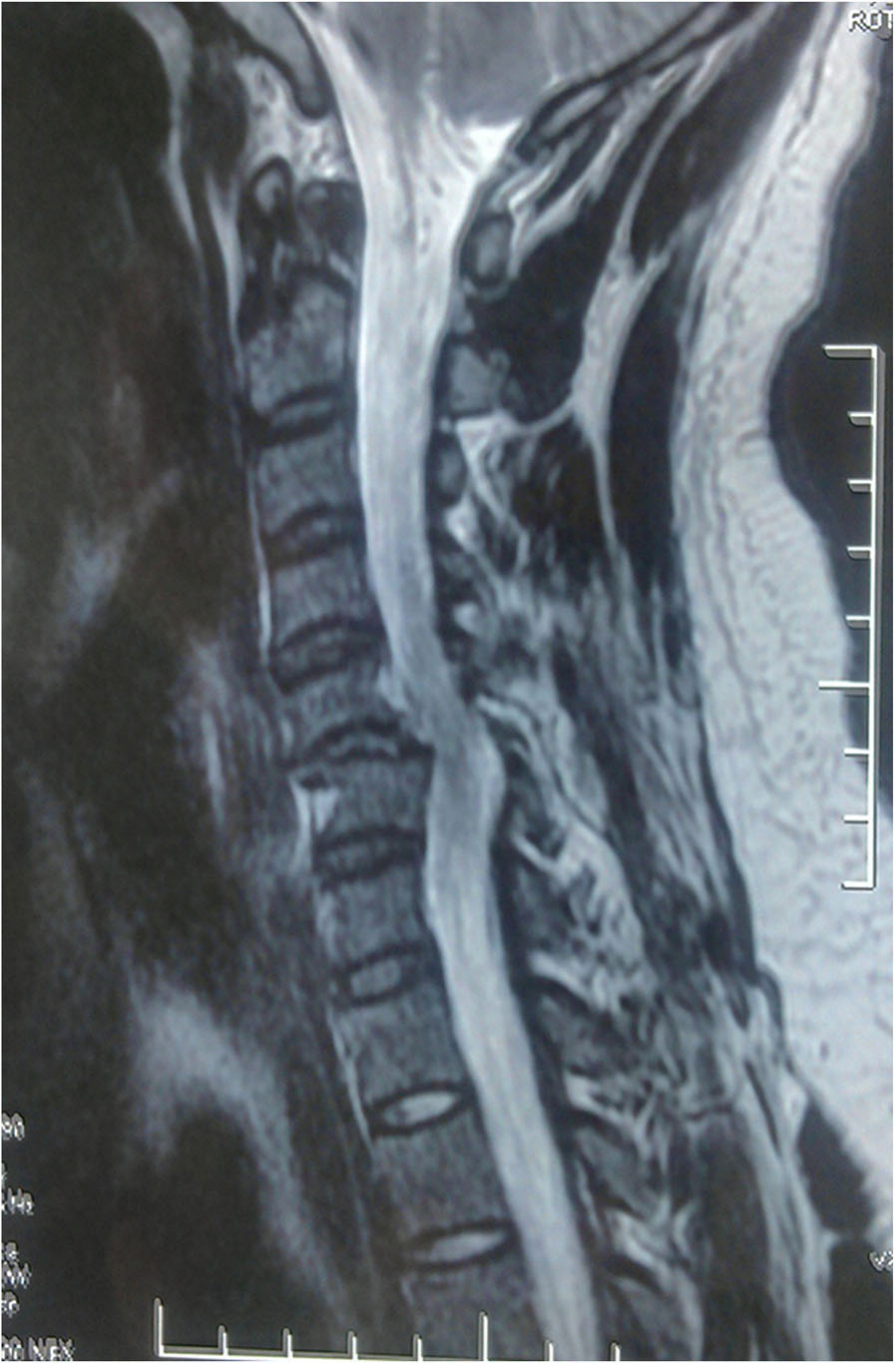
Preoperative magnetic resonance imaging: C5/6 disk rupture and extrusion and severe cord compression with increased signal changes
